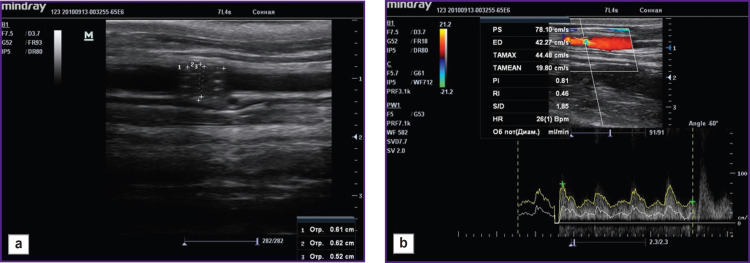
Ultrasound control of the stent-graft patency: (a) imaging of the stent-graft struts; (b) confirmation of the blood flow through a stent-graft
